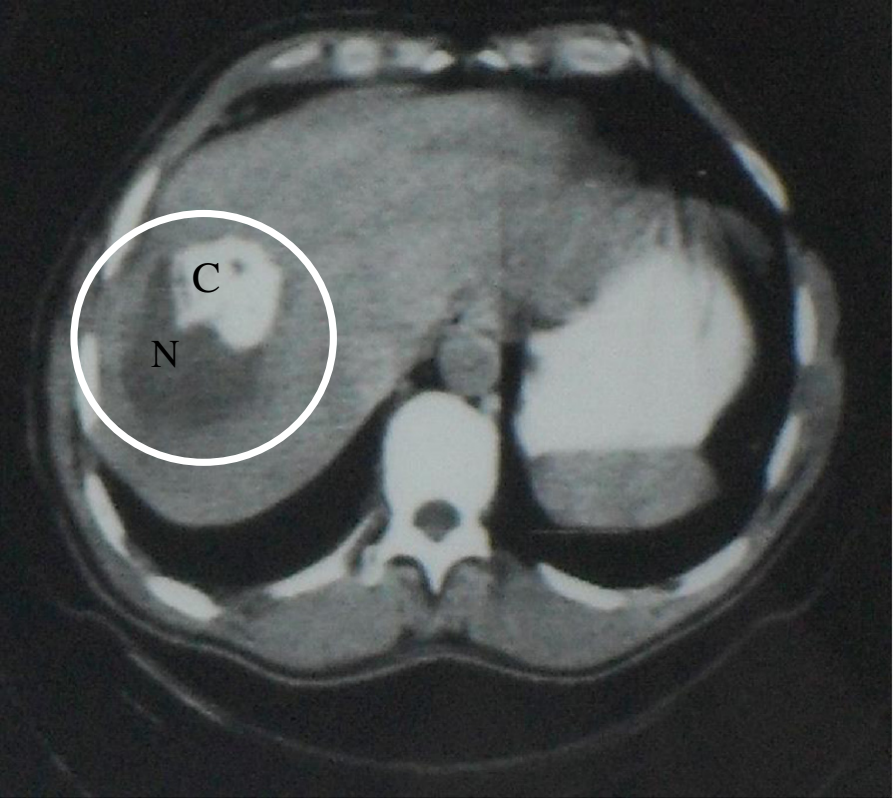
Abdominal CT Scan (1982): alveolar echinococcosis heterogeneous lesion of the right lobe of the liver, including a large necrotic area (N) and a calcified area (C).
